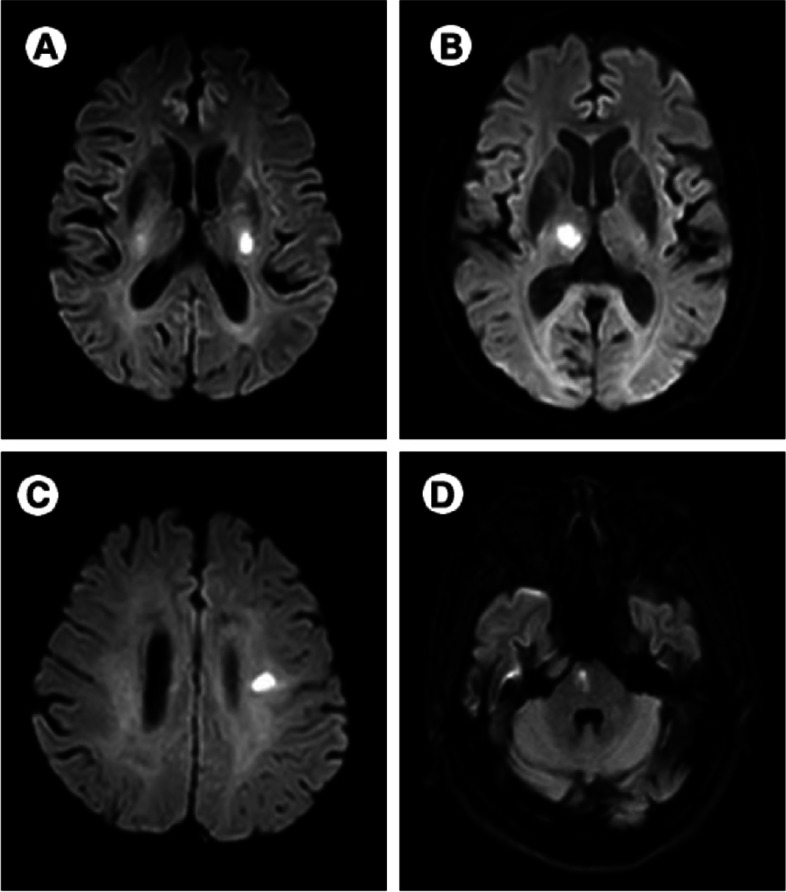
Examples of the four different features of MRI-confirmed small vessel diseases: lacune (A), cerebral microbleeds (B), enlarged perivascular spaces (C), and white matter hyperintensities (D)
